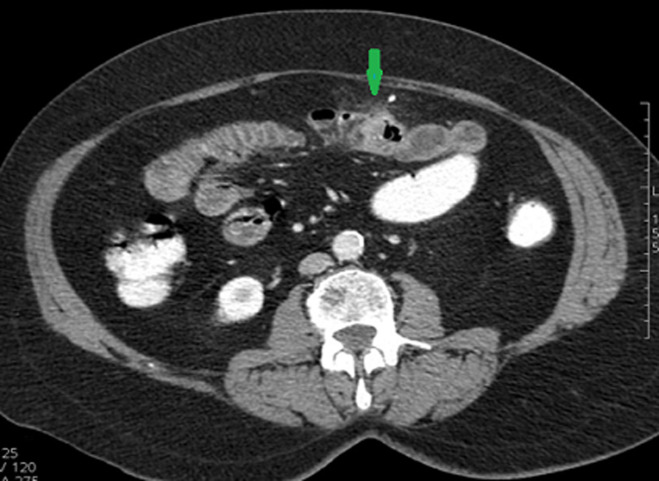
CT-scan (axial view) showing two large jejunal diverticula with wall thickening of the affected segment and the infiltration of the surrounding mesenteric fat
